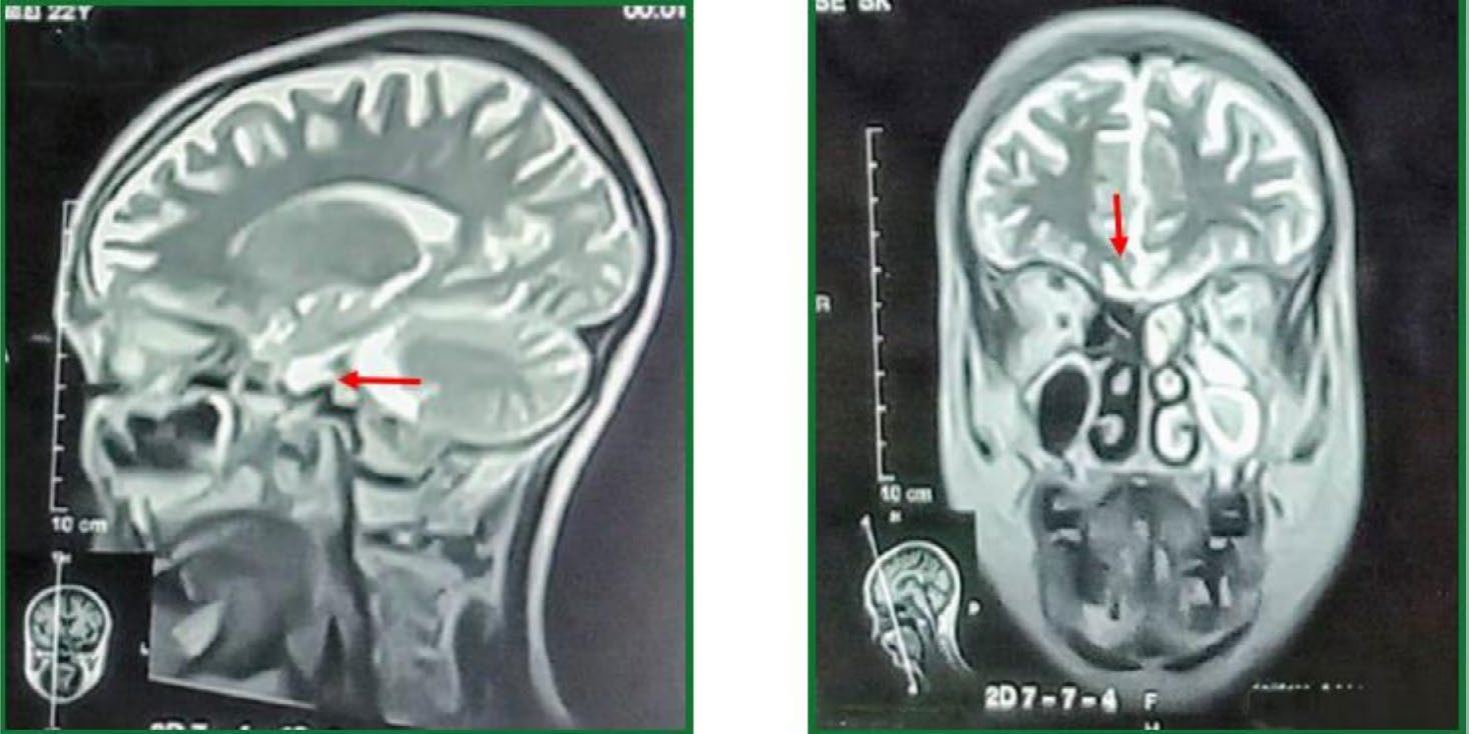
Sagittal and coronal MRI sections of the brain demonstrate a normal pituitary gland (red arrow, left) and well‐developed olfactory bulbs and olfactory sulci (red arrow, right).
